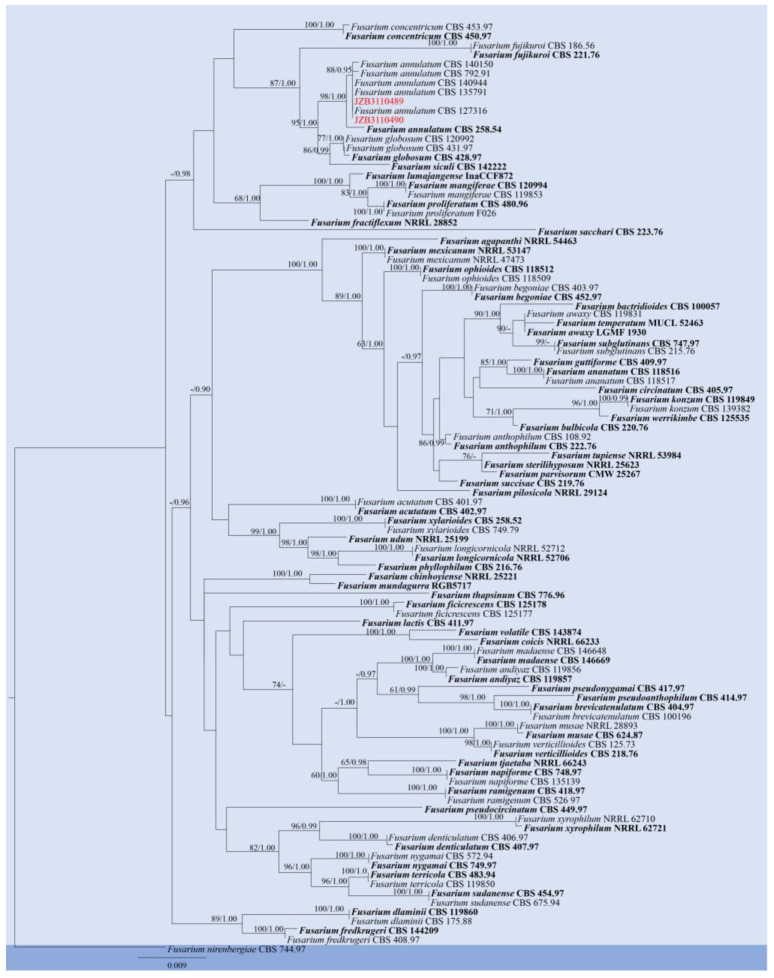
Phylogenetic tree generated by maximum likelihood (ML) analysis of combined *tef 1-α* and *rpb2* sequence data of *Fusarium* species. The tree is rooted with *Fusarium nirenbergiae* (CBS 744.97). The matrix has 421 distinct alignment patterns, with 3.70% of characters or gaps undetermined. Estimated base frequencies are as follows: A = 0.263854; C = 0.251445; G = 0.251382; T = 0.233319. Substitution rates: AC = 1.435916; AG = 6.155181; AT = 1.375277; CG = 0.854277; CT = 13.787190; GT = 1.000000. Gamma distribution shape parameter: α = 0.814503. ML bootstrap support values ≥ 60% and Bayesian posterior probabilities (BYPP) ≥ 0.90 are given near the nodes. The scale bar indicates 0.009 changes. The ex-type strains are in bold, and newly generated sequences are in red.
